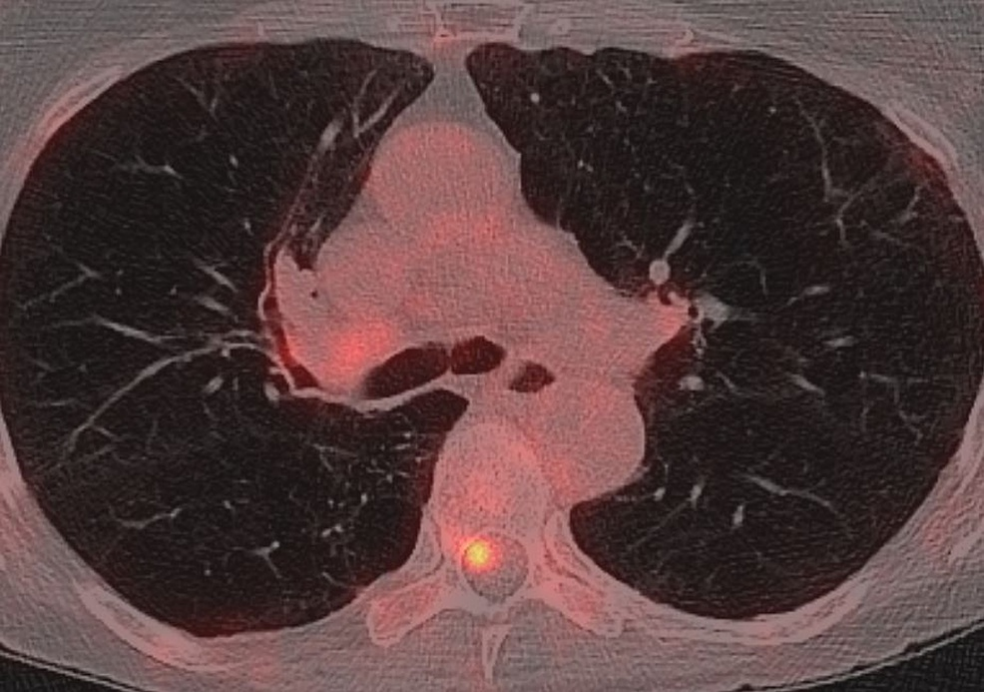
Axial PET-CT scan (tracer 18F-fluorodeoxyglucose) showing intraspinal tracer uptake at the level of the seventh thoracic vertebra, clearly separated from the lung primary. PET-CT, positron emission tomography-computed tomography
